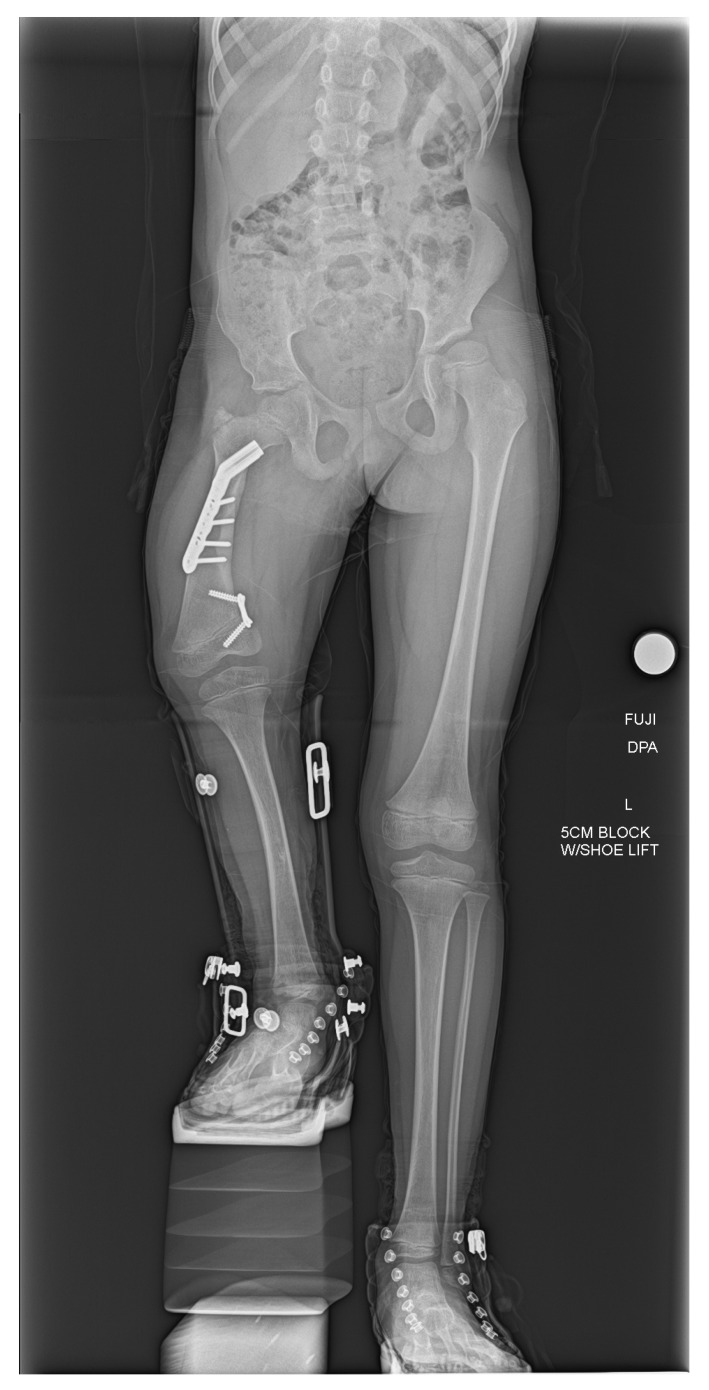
AP bilateral lower extremity radiograph post-SUPERhip procedure. Right femoral osteotomies are healing appropriately, and hardware is intact and well tolerated.
